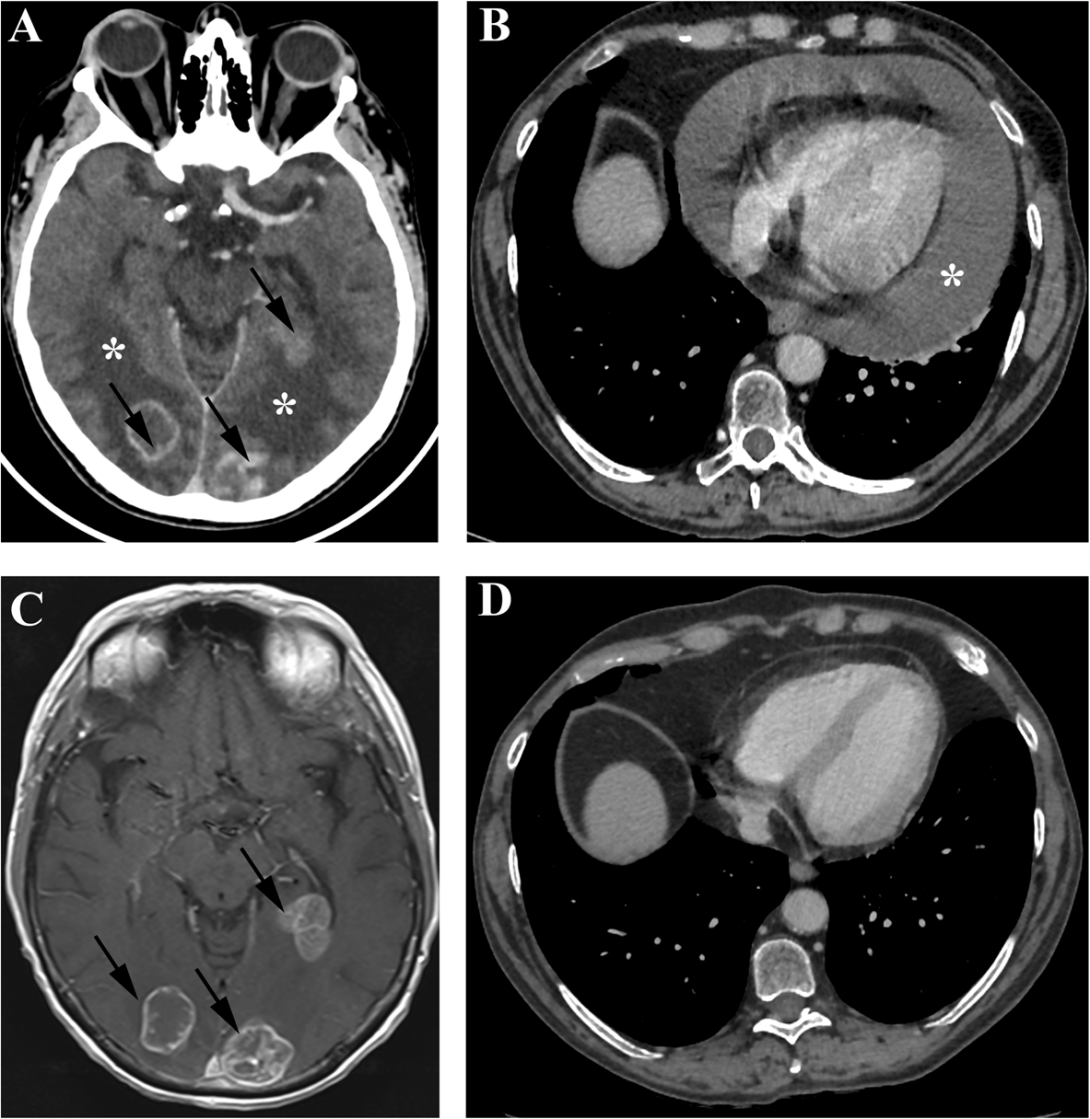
Cerebral and chest imaging of patient 1. a Axial cerebral CT section displaying multiple brain lesions (arrows) with perilesional oedema after the 4^th^ infusion of nivolumab. Brain lesions decreased in size while perilesional oedema was significantly increased. b Axial chest CT imaging showing cardiomegaly with pericardial effusion (asterisk) after the 4th infusion of nivolumab. Note the absence of radiological evidence of pericardial or pleural cancer involvement, dilatation of the right cavities. c Axial gadolinium-enhanced T1-weighted MRI at baseline before the initiation of nivolumab. d Axial chest CT imaging at baseline before the initiation of nivolumab
